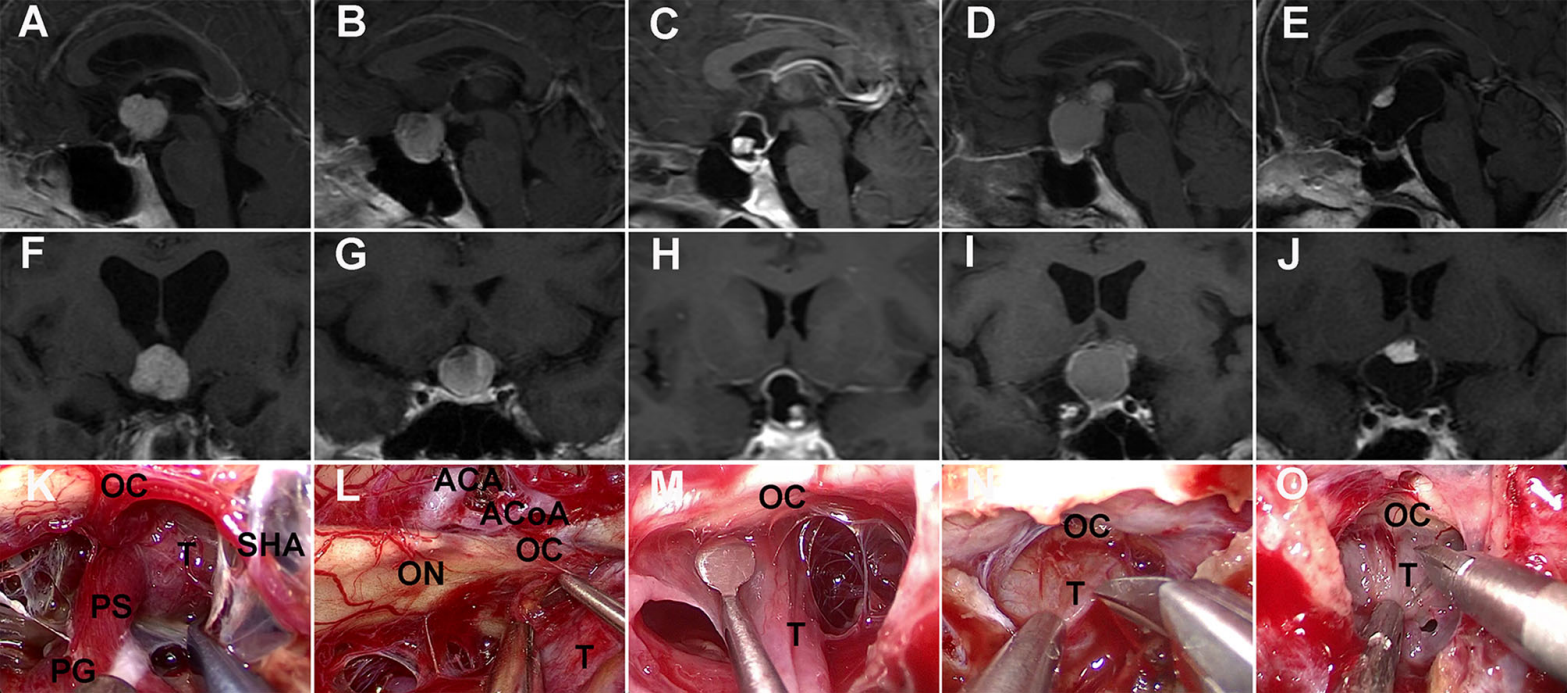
Adhesion strength between craniopharyngioma and optic nerves was intraoperatively evaluated. (K, L, M) No or loose adhesion. Contrast-enhanced T1-weighted MRI scans (A, F) showing an intrinsic third ventricular solid tumor compressing forward the optic chiasm. Intraoperative view (K) revealing that the proximal part of the pituitary stalk extending from the gland could be identified as intact and at a normal size before tumor resection. Preoperative MRI scans (B, C, G, H) showing a sellar-suprasellar/suprasellar cystic-solid tumor stretching upward the optic chiasm. Surgical view (L, M) showing that the tumor can be easily separated from the optic nerves by dissector. (N, O) Tight adhesion. Preoperative MRI scans (D, E, I, J) showing sellar-suprasellar cystic-solid tumors displacing the optic chiasm. Intraoperative video-captured photographs (N, O) showing tight adhesion between the tumor and the optic apparatus needing sharp dissection using scissors. OC, optic chiasma; ON, optic nerve; T, tumor; PG, pituitary gland; PS, pituitary stalk; SHA, superior hypophysial artery; ACA, anterior cerebral artery; ACoA, anterior communicating artery.
